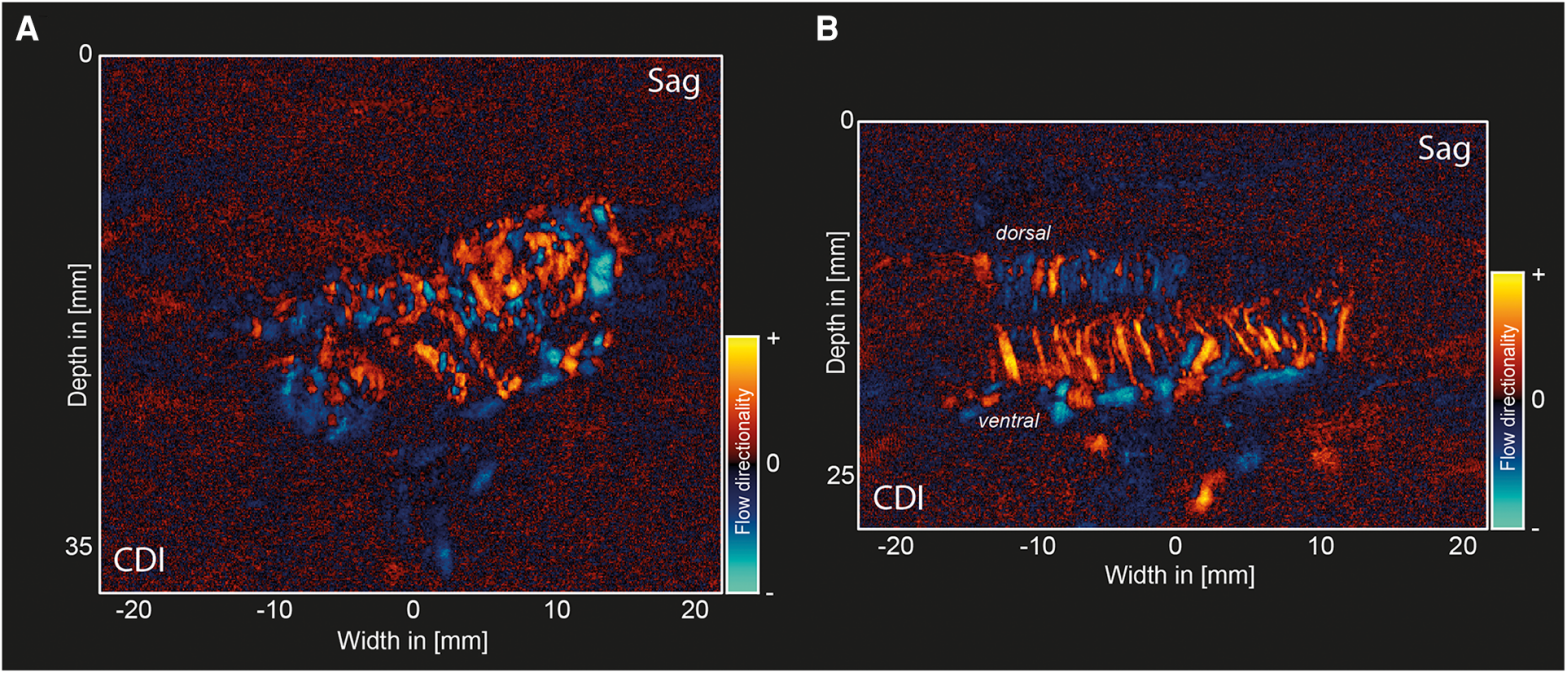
Color Doppler images (CDIs) pre-and post-resection of a hemangioblastoma. (A) Pre-resection, sagittal CDI of the same plane as in Figure 3A, The color axis depicts flow directionality with positive values indicating flow *towards* the transducer, and negative values indicating flow *away* from the transducer. (B) Sagittal CDI of the same plane previously shown in Figure 4B. CDI, Color Doppler Image.
